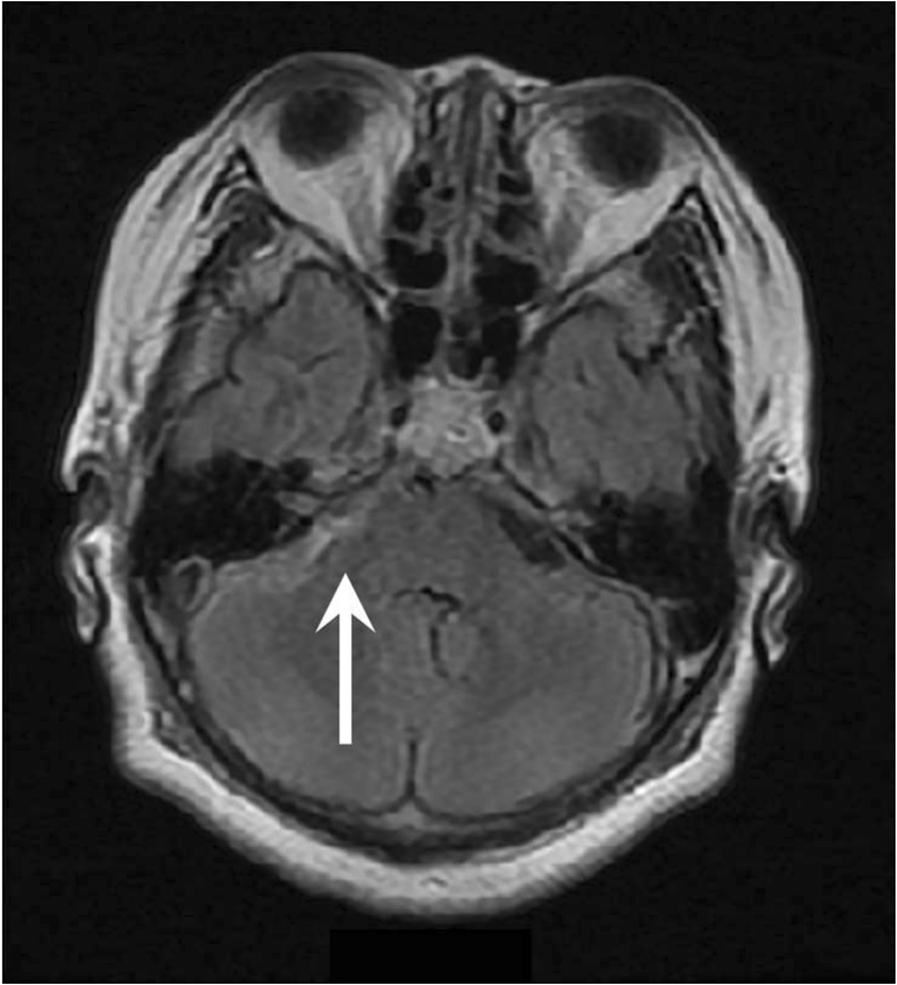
T2-weighted magnetic resonance imaging of the brain showing a subarachnoid hemorrhage at the right cerebellopontine angle (arrow)
